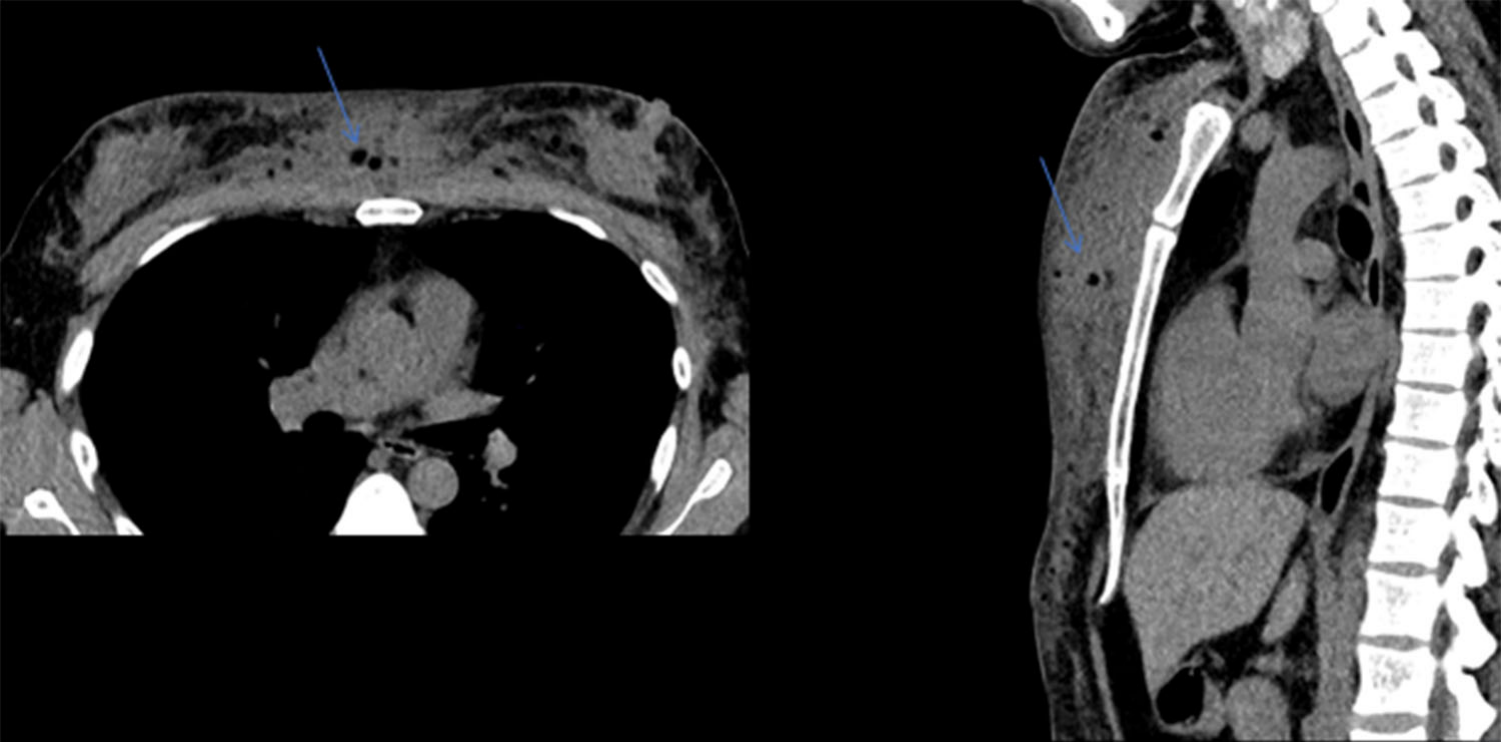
Chest wall migration of mineral oil incidentally seen on a CT scan in a 41-year-old transgender woman with a history of breast augmentation with free mineral oil self-injections (arrows)
